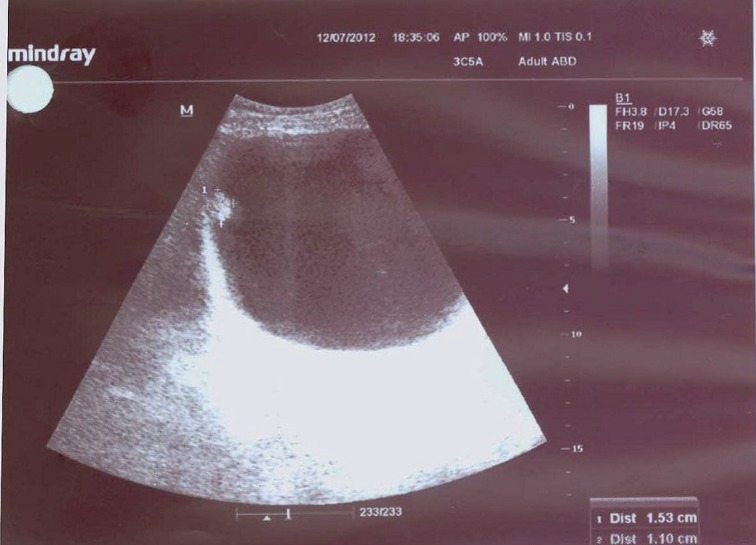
Bladder ultrasonography showing the soft-tissue lesion in the right bladder wall of a girl aged 17-years.
